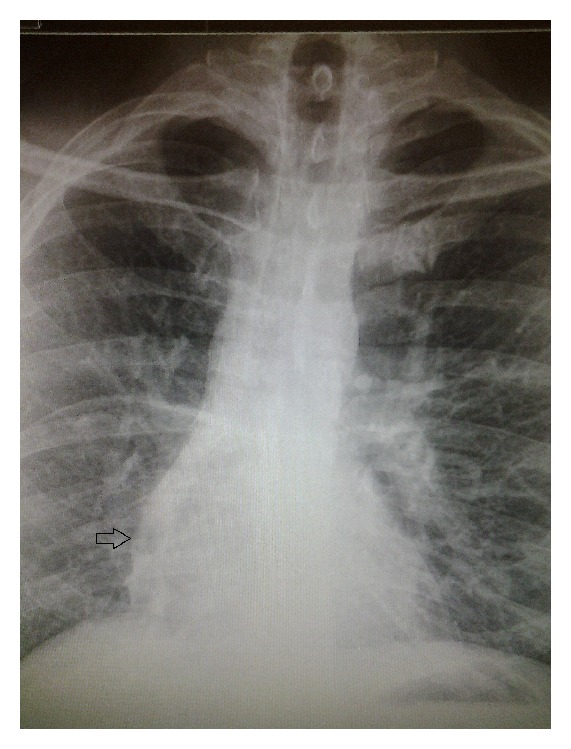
PA chest X-ray showing shadow representing anomalous pulmonary vein coursing alongside right heart border caudally.
